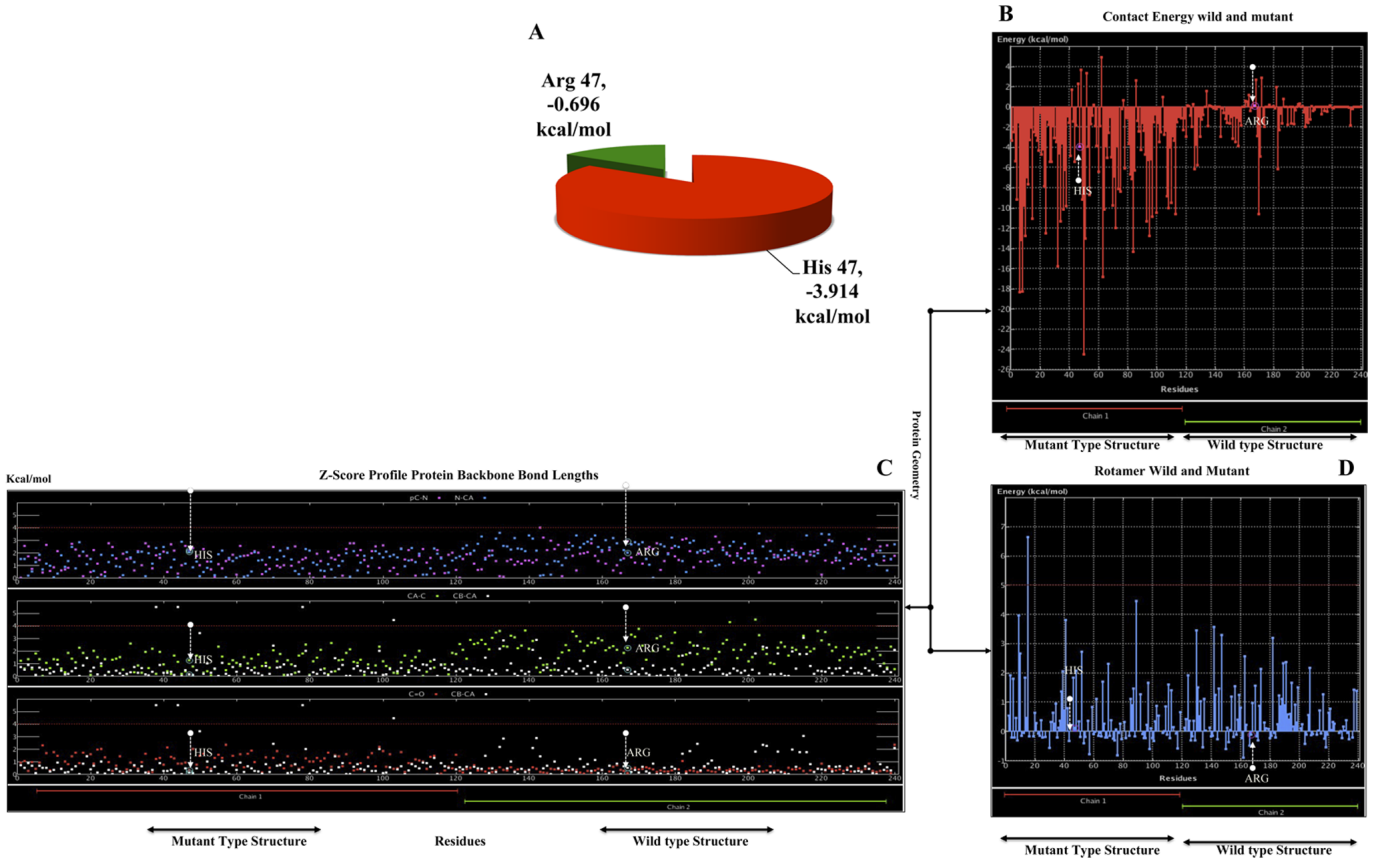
Contact energy and protein geometry of the TREM2 domain. A) Pie chart showing the energy significance for wild-type and mutant residues of Arg⇒His47, i.e., wild-type Arg47 (green) −0.696 kcal/mol and His (red) mutant type −3.914 kcal/mol. B) The constructed TREM2 domains for wild-type and mutant positions of the amino acid residues are shown on the x-axis, while the contact energies are shown on the y-axis. The chain 1 (red) mutant type structure of residue His47 energy was shown on the y-axis is indicated by the arrow shown in the downstream region (white), but chain 2 (green) of wild type residue His47 energy is shown as the x-axis and is indicated by the arrow shown in the upstream region (white). The trends in the variation of the contact energy in most of the parts of the TREM2 domain were in good agreement with that of the X-ray structure of TREM2. C) The rotamer wild type and mutant structure residue positions are shown on the x-axis, although the contact energies are shown on the y-axis. The chain 1 (red) mutant type structure of amino acid residue His47 energy was shown as the y-axis indicated by the arrow shown in the upstream region (white), but chain 2 (green) of wild-type residue His47 energy was shown as the x-axis indicated by the arrow shown in the downstream region (white). D) The protein backbone of both mutants was shown in red (chain-1), and the wild type from is shown in green, with a bond length that is based on the Z-score profile of the protein geometry. The distribution of three portions is given: 1) [PC-N (pink), N-CA (blue), 2) CA-C (green), CB-CA (white), and 3) C = O (red), CB-CA (white).
